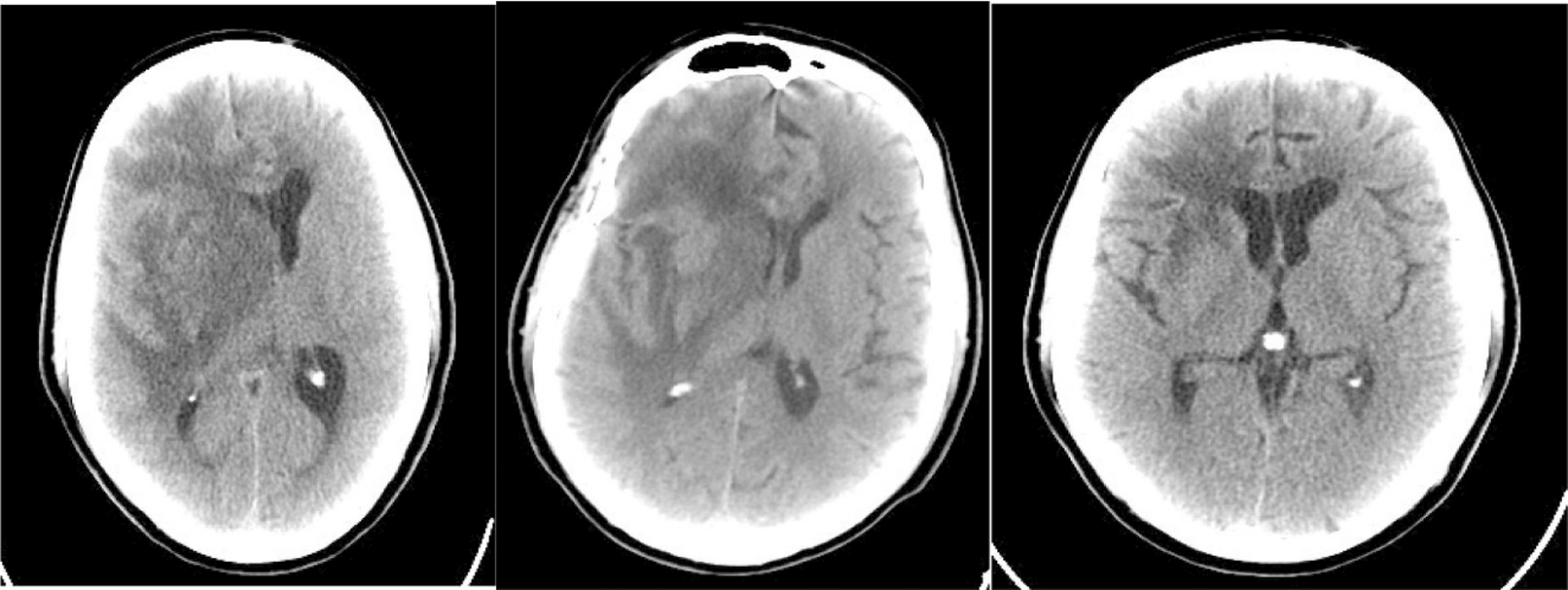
Brain CT involvement at the time of the diagnosis and response to the treatment. Left: Diffuse Fronto‐temporal vasogenic edema at presentation. Middle: After two sessions of plasma exchange in week one. Right: One week after first course of systemic chemotherapy.
